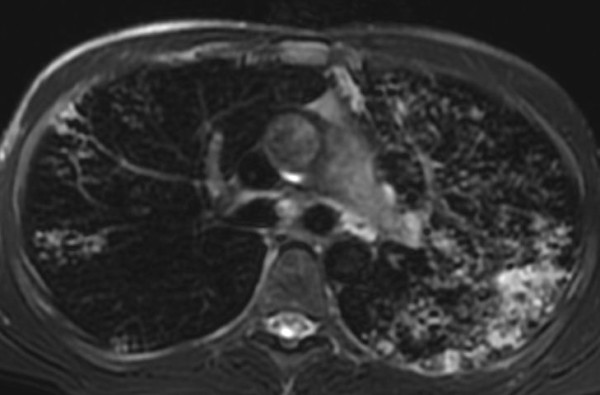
Same patient reported in Fig.2: 28 year-old man with pulmonary tuberculosis, unenhanced MRI identifies the same features as well as HRCT in figure 2.
